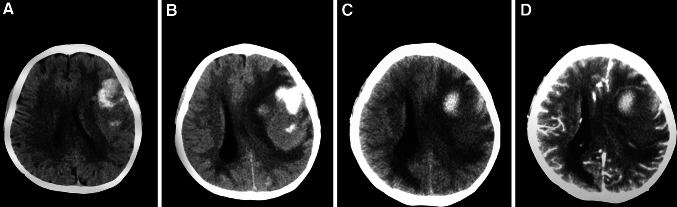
A case of subacute progressing stroke. The patient was admitted to our hospital because of dysphagia, hemiparalysis, and conscious disturbance. a CT on hospital admission showed left parietal and frontal intracerebral haemorrhage. After the initial treatment, the patient regained consciousness. b 10 days after hospital admission, the patient became lethargic, and repeated CT showed an increased mass effect and midline shift, with enlargement of the perihaematomal oedema. Stronger osmotic therapy was applied immediately and the patient’s consciousness improved. c Repeated CT at 17 days post hospital admission showed that the initial haematoma shrank further, the density of the brain oedema decreased, and the mass effect alleviated modestly. d An enhanced CT at 17 days showed no ring-enhancement, eliminating the tension haematoma
